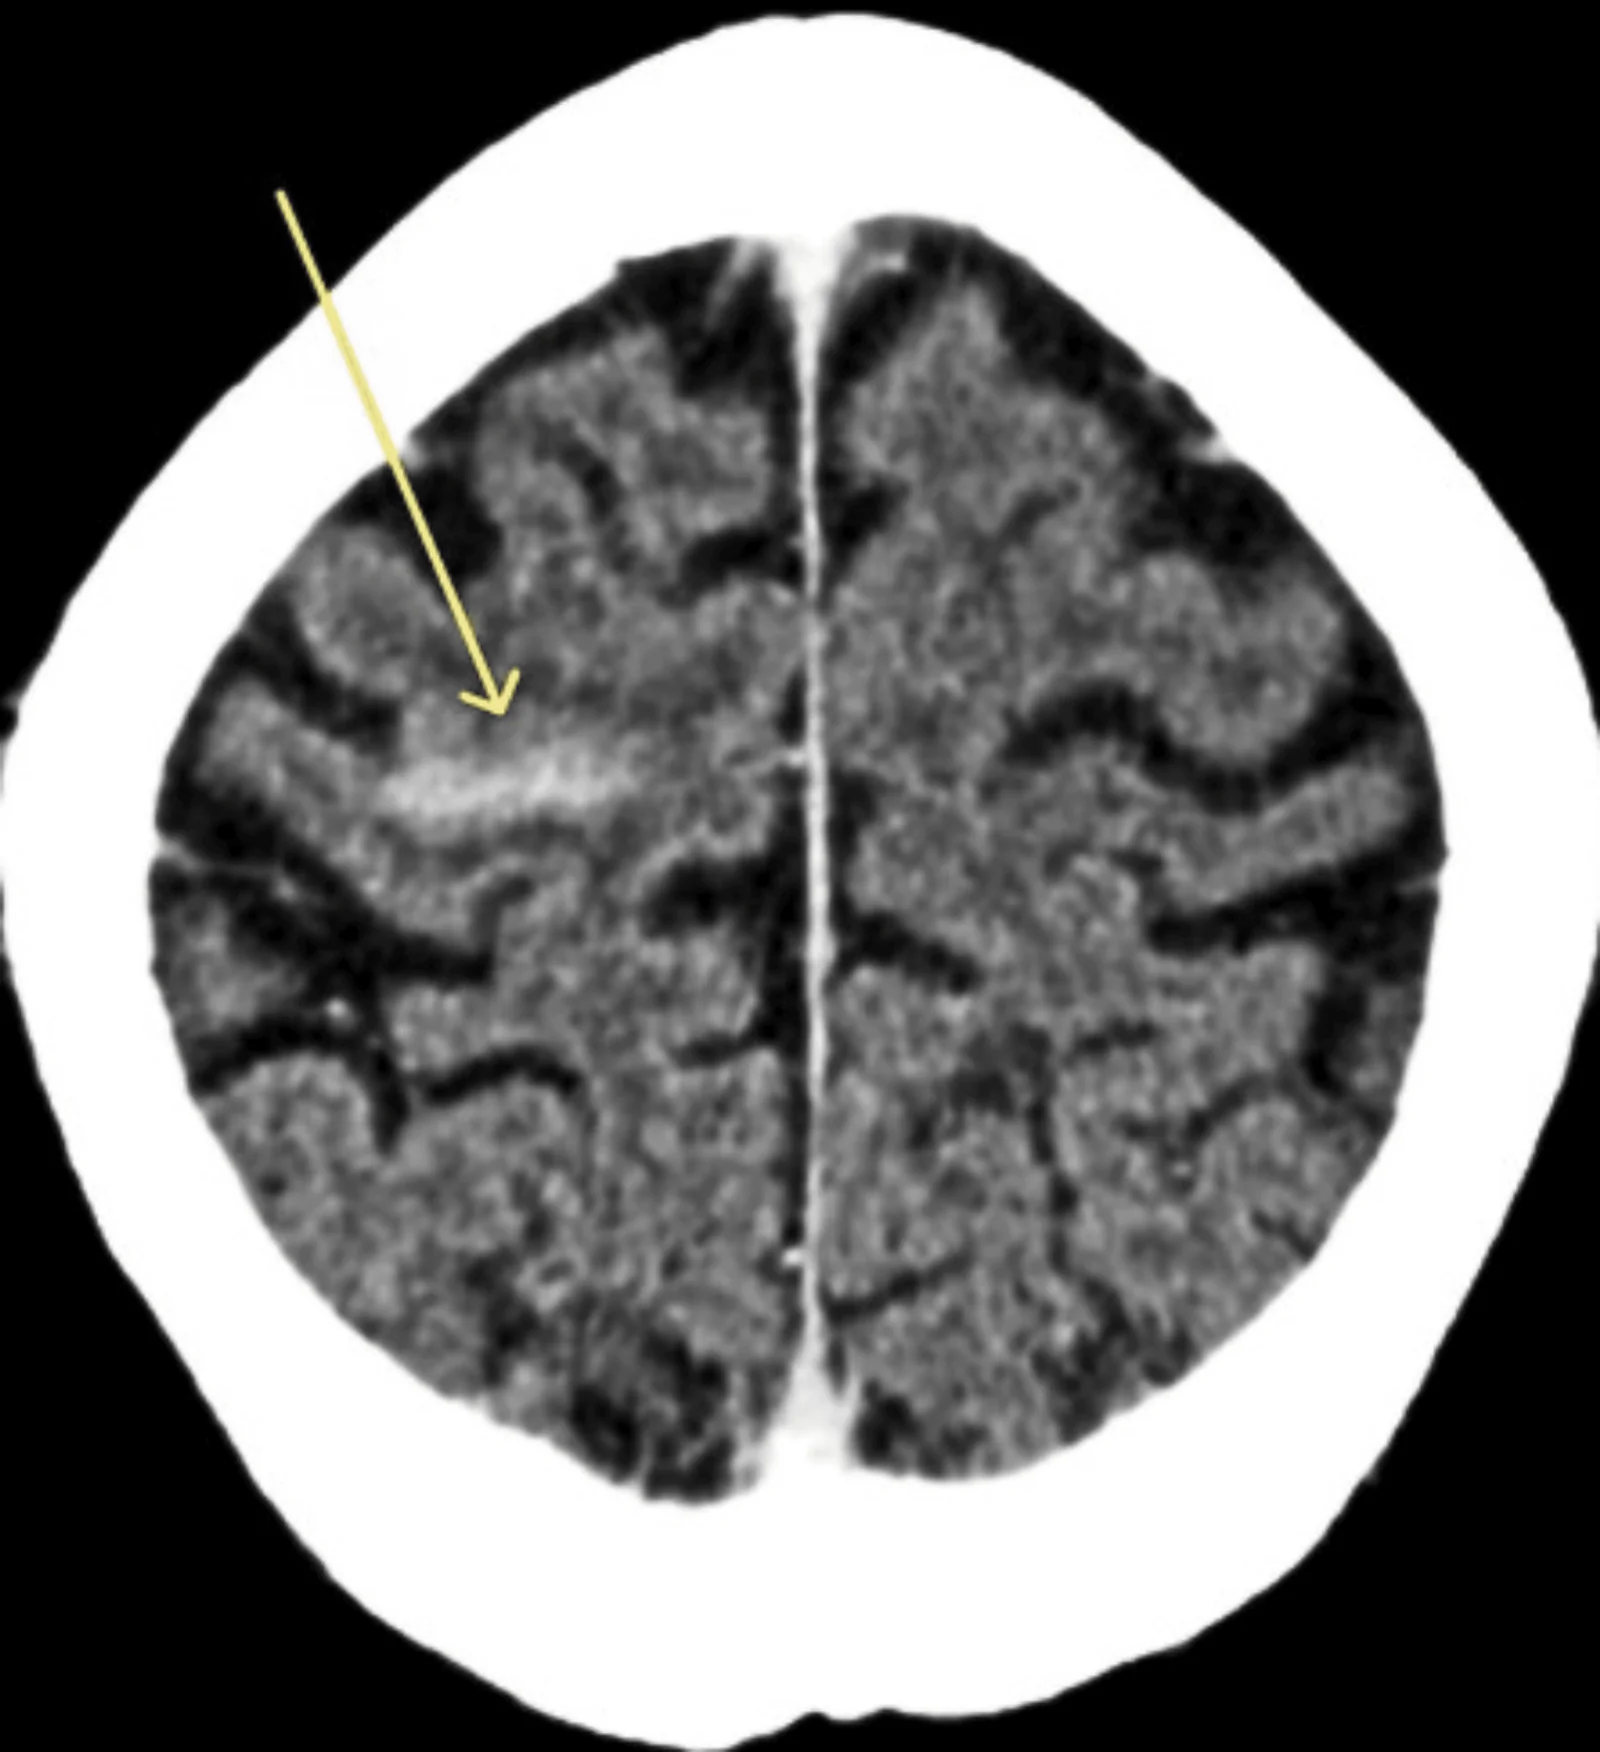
Axial contrast-enhanced CT of the head on Day 3 demonstrating acute right frontal SAH (yellow arrow), with unchanged right frontal lobe hypoattenuation consistent with a chronic infarct CT: computed tomography; SAH: subarachnoid hemorrhage
